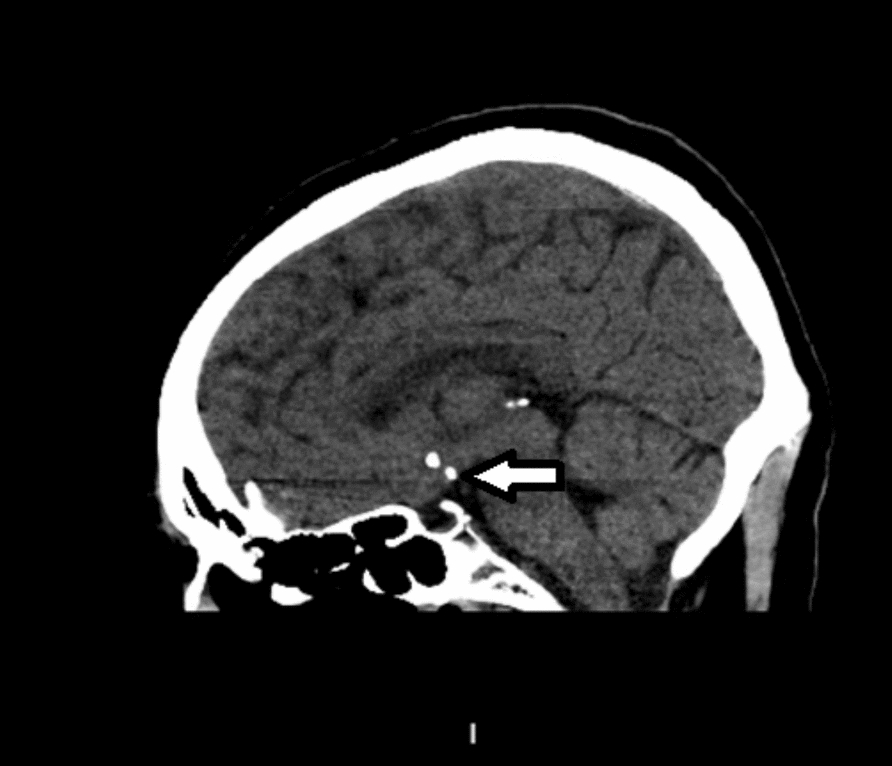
Sagittal Computed Tomography (CT) image of the brain highlighting involvement of the hypothalamic-suprasellar area with abnormal attenuation, consistent with granulomatous disease
